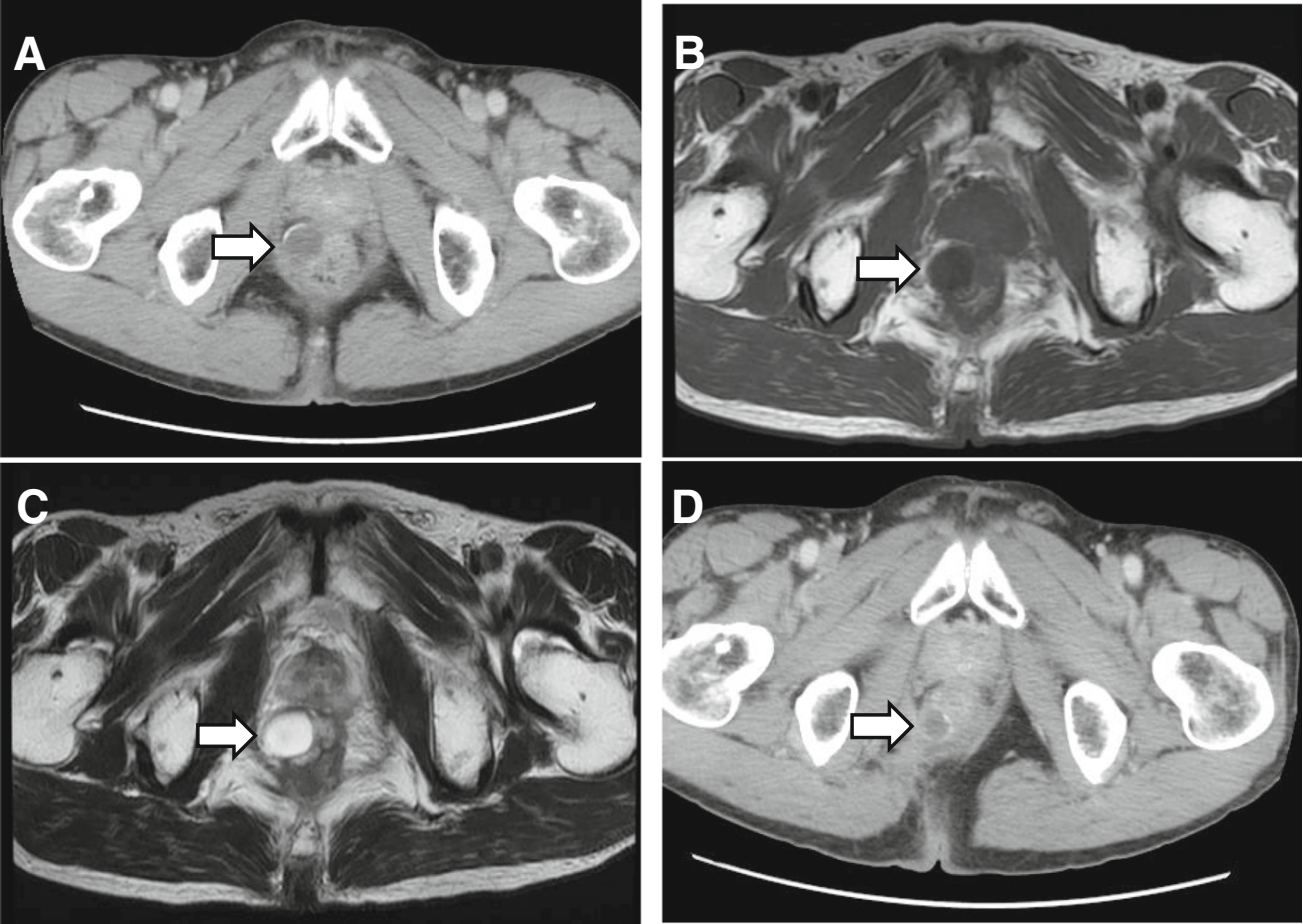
Abdominal (a) computed tomography images, b T1-weighted magnetic resonance images, and (c) T2-weighed magnetic resonance images prior to the first operation. A submucosal cystic lesion without enhancement of the capsule was seen on the right side of the lower rectal wall. It contained homogeneous fluid with calcification of the capsule. d A similar tumor was detected by computed tomography 14 months after the first operation
